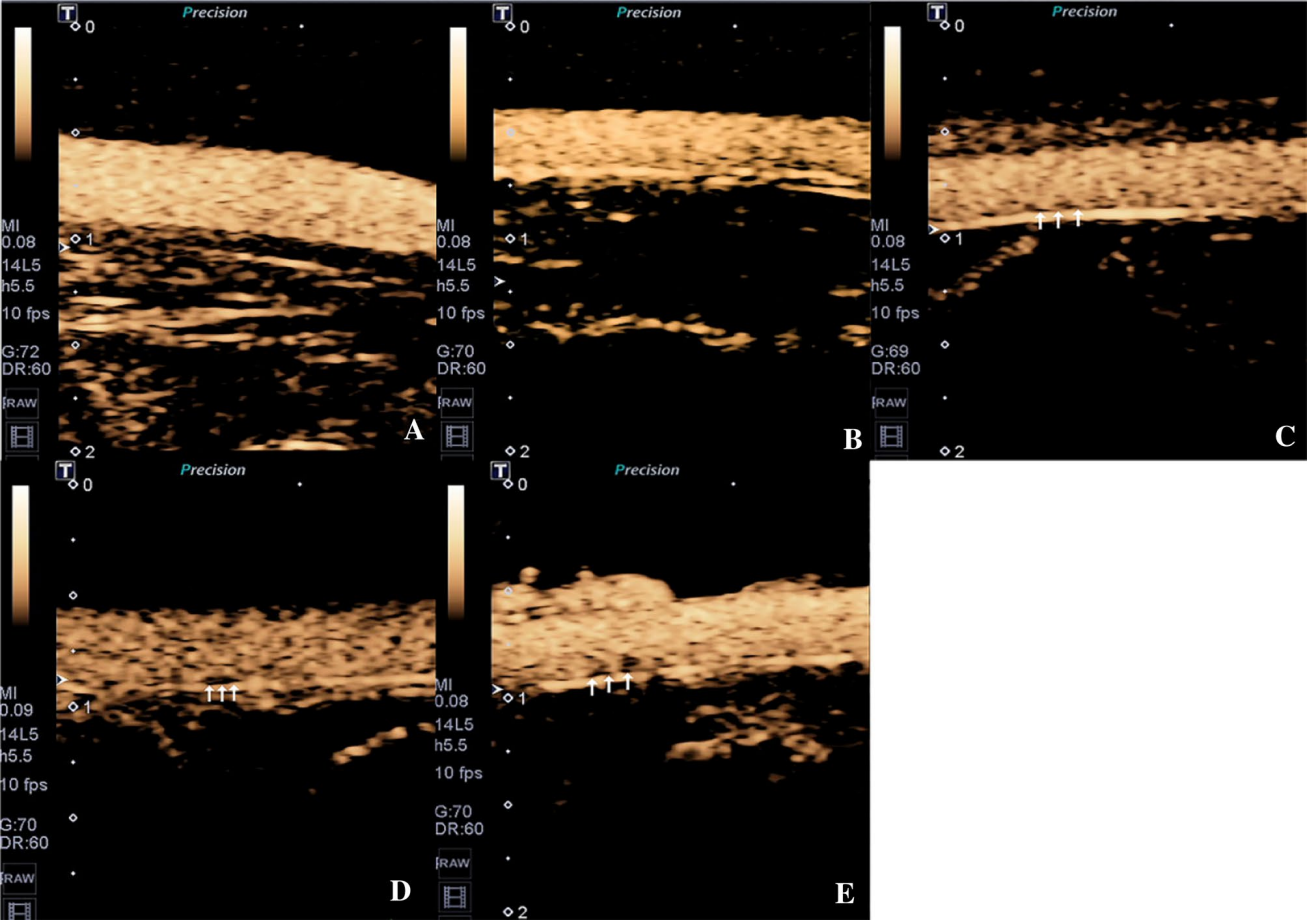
SonoVue ultrasonic contrast images of abdominal aorta of rabbits in different groups, five rabbits in each group. (A) Control group; (B) Week-4 group; (C) Week-8 group; (D) Week-12 group; (E) Week-16 group. The plaque on the wall was indicated by the arrows.
